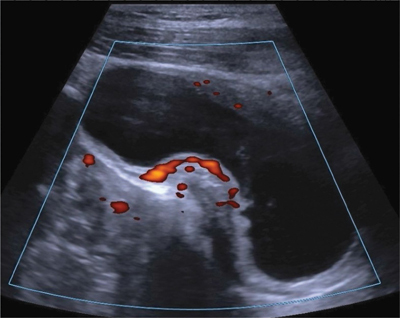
A 23-year-old patient. Color Doppler ultrasound in the sagittal section showing the ‘hourglass’ appearance due to the localised hypervascular bladder wall thickening.
